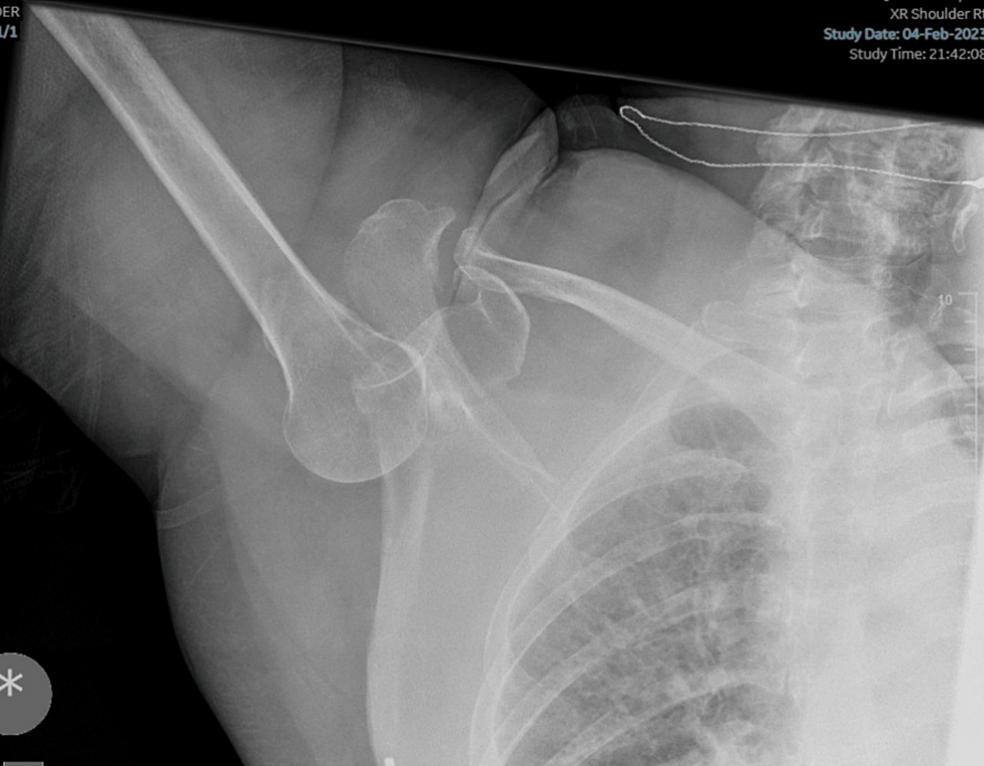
An anteroposterior X-ray showing the humeral head in an inferior subglenoid position with a greater tuberosity fracture.
